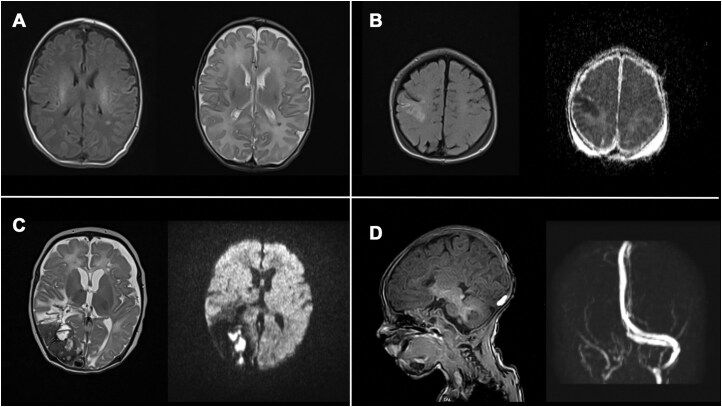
Pathological MRI findings in CHD patients. Preoperative (A, B) and postoperative MRI (C, D). (A) Periventricular WMI on T1 and T2, left parietal, in a newborn with UVH. (B) Subacute infarction in the right hemisphere with involvement of the precentral and postcentral gyrus on T2 and ADC (apparent diffusion coefficient image) in a newborn with D-TGA after balloon atrial septostomy. (C) Extensive colliquation necrosis of the posterior part of the right cerebral hemisphere with a large area of haemorrhages on T2 and ADC in a HLHS patient after hybrid and secondary Norwood I operation. (D) Haemorrhage in the area of the confluens sinuum and underlying sinus vein thrombosis of the right transverse sinus on T1 and MR-venography in a HLHS newborn after hybrid stage I
